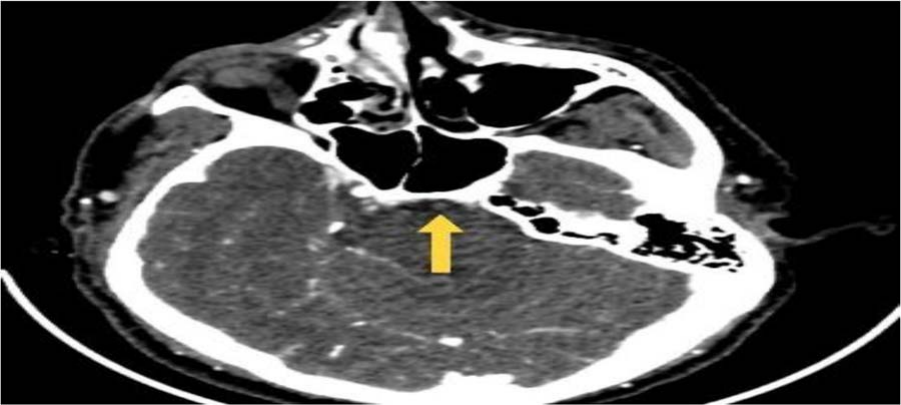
Axial CTA image of the brain demonstrating abrupt loss of contrast opacification at the proximal basilar artery (arrow), consistent with acute basilar artery occlusion.
